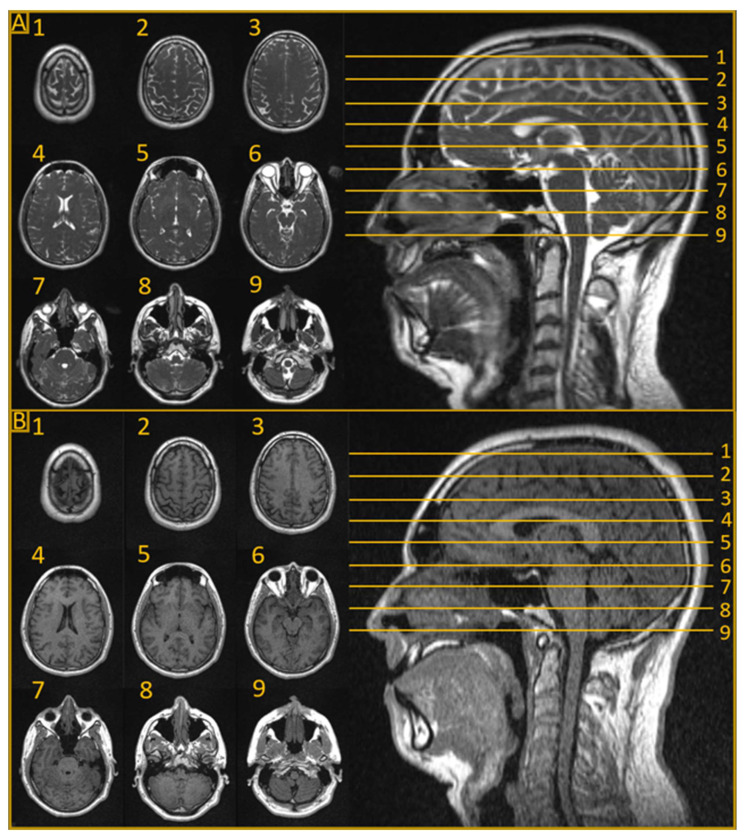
Magnetic resonance imaging (MRI) sequences on a 0.35 T combination MRI and linear accelerator (MRL) without contrast. (A) A true fast imaging with steady-state free precession (TRUFI) sequence of a healthy brain. The in-plane resolution and acquisition time for the TRUFI sequence were 1.5 mm and 1 min and 40 s, respectively. The TRUFI sequence provides contrast that is a combination of T_1_ and T_2_ weighting and is the sequence used for planning on the 0.35 T MRL. (B) T_2_ fluid-attenuated inversion recovery (FLAIR) sequence of a healthy brain. The in-plane resolution and acquisition time for the FLAIR sequence were 0.75 mm and 12 min and 6 s, respectively. Both sets of images (FLAIR and TRUFI) have a slice thickness of 1.5 mm and were acquired using a six-channel phased-array receiver head coil.
